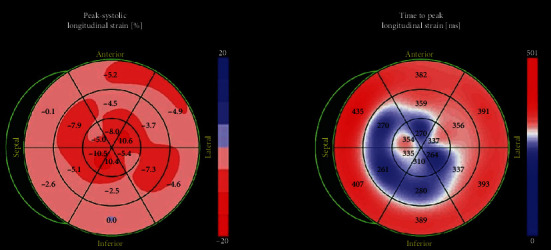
Echocardiographic polar map highlighted a decrease in global longitudinal strain.
